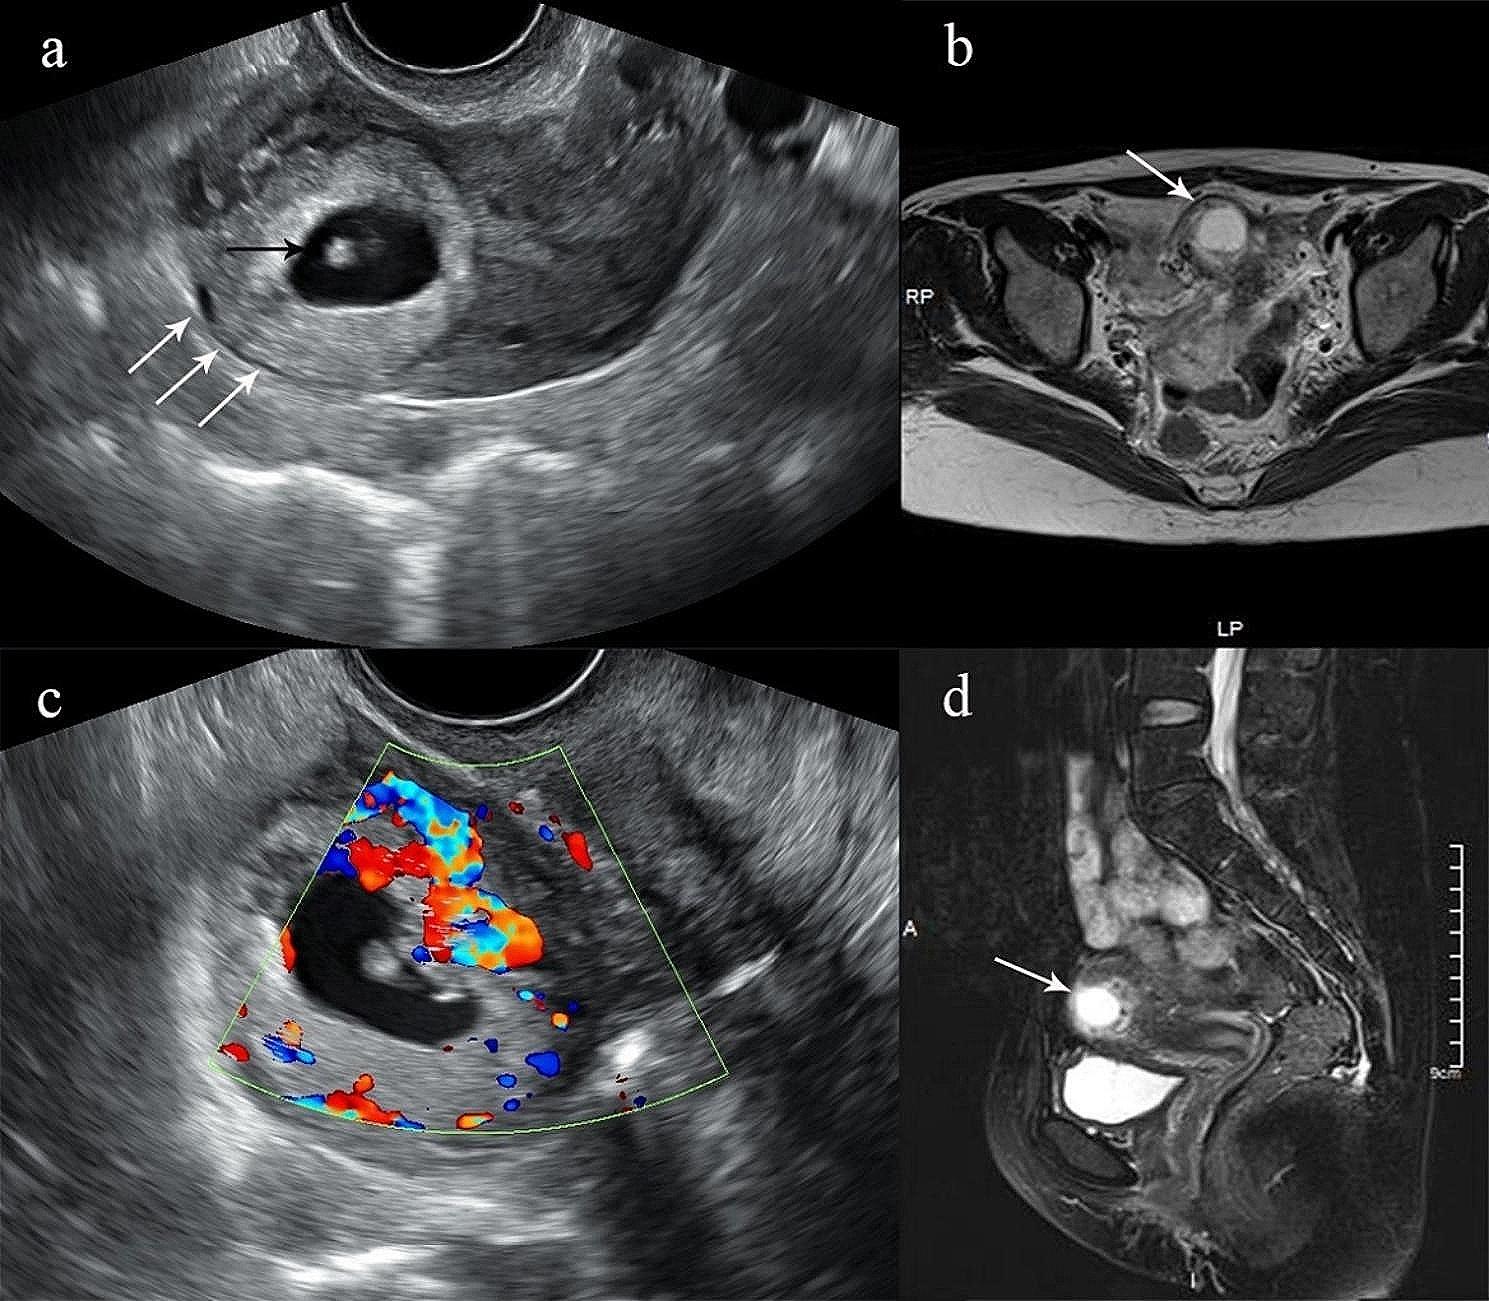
(a) Transvaginal ultrasound revealed a gestational sac (black arrow) embedded in the fundal myometrium near the right cornu of the uterus. The endometrial lining was indistinct and the uterine fundal serosa was thin, with a residual myometrial thickness of 2 mm (white arrow). (c) Color Doppler ultrasound demonstrated increased peritrophoblastic blood flow. (b) Magnetic resonance imaging (MRI) in the coronal plane demonstrated a gestational sac with intermediate to low echogenicity extending beyond the uterine contour, associated with marked myometrial thinning and indistinct demarcation from the uterine wall (white arrow). (d) MRI in the sagittal plane revealed an irregularly shaped uterus, with discontinuous endometrium and junctional zone, and a cystic lesion with high signal intensity at the uterine fundus (white arrow)
